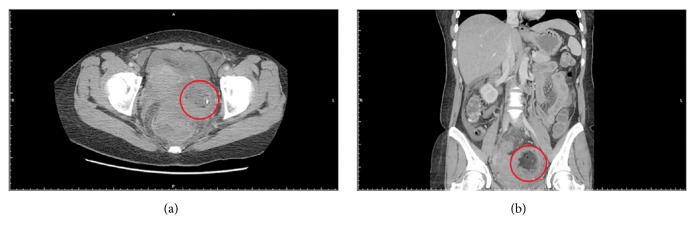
Abdominal CT survey. CT arranged in ER showed a mixed density mass about 4*∗*4(cm) with calcifications but discontinuous border over the left adnexa ((a) axial view of abdominal CT; (b) coronal view of abdominal CT).
